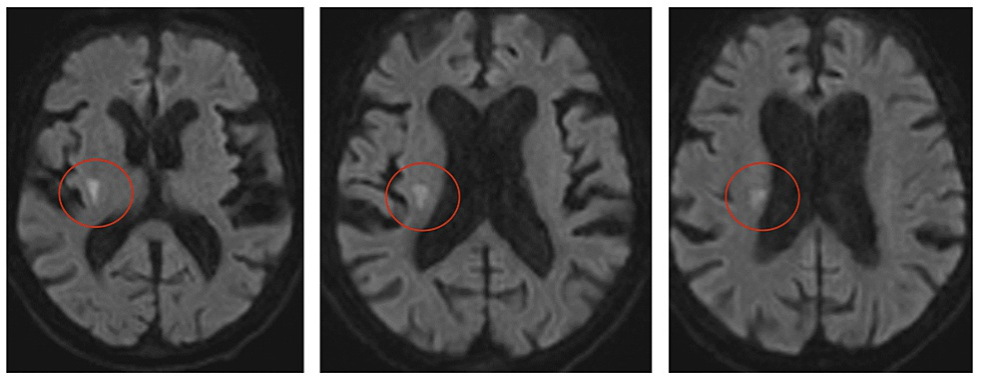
Brain MRI DWI sequence showing acute ischemic infarct (red circles) starting in basal ganglia and extending up to the right coronal radiata MRI: magnetic resonance imaging; DWI: diffusion-weighted imaging
